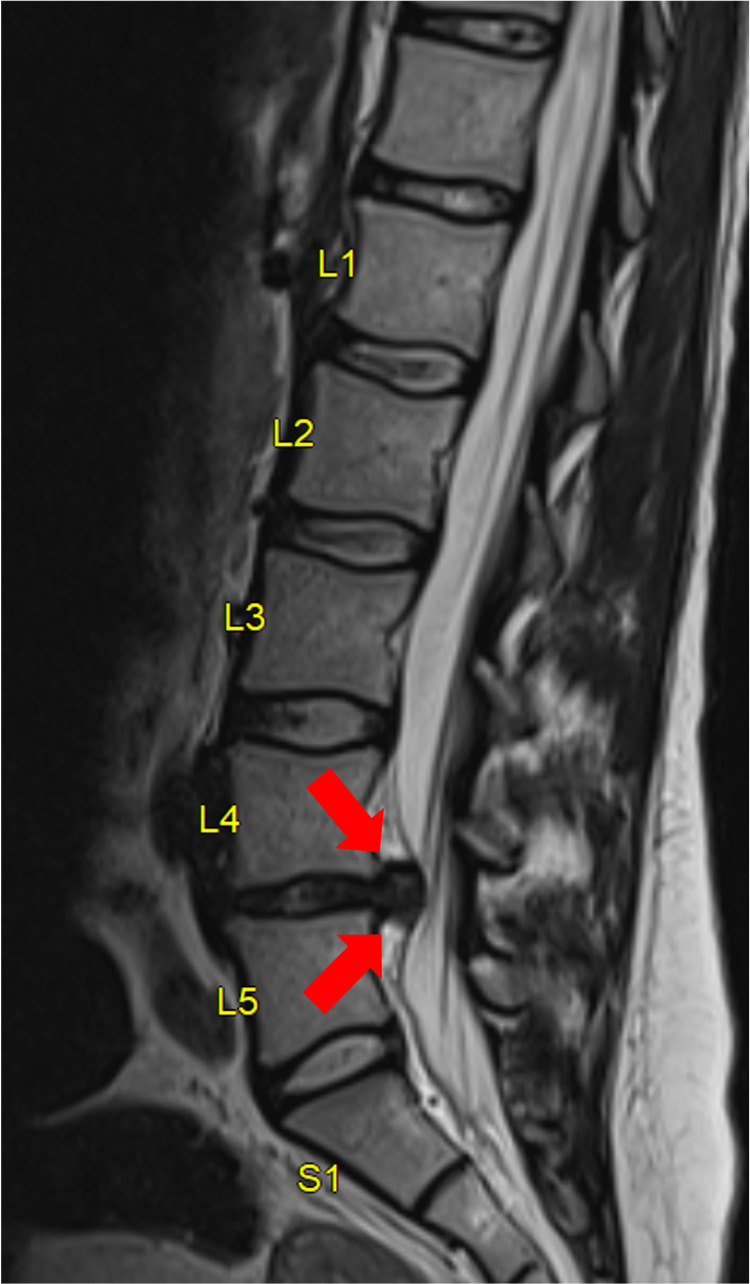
Sagittal MRI of the lumbar spine. Large paracentral L4-5 disc herniation (red arrow).
